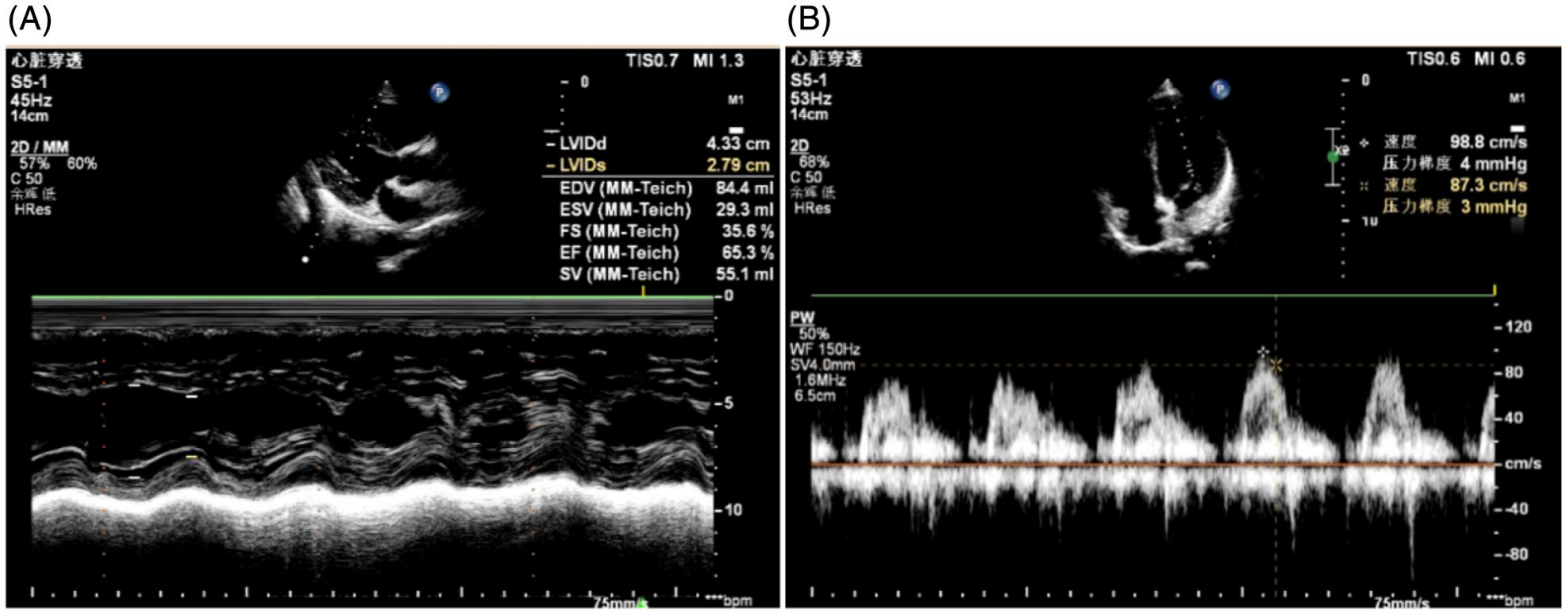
Transthoracic echocardiography with Doppler. (A) Demonstrates left ventricular ejection fraction (LVEF) of 65.3%. (B) Shows mitral inflow velocities—E‐wave 98.8 cm/s, A‐wave 87.3 cm/s, E/A ratio = 1.13.
